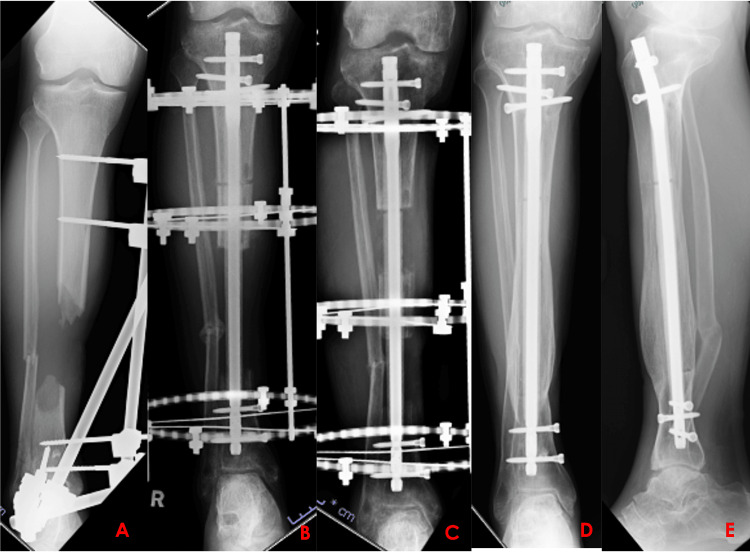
Serial radiographs of a case of bone transport over nail. (A) A trauma film shows significant bone loss of the tibia diaphysis. (B) Following an unhealthy bone resection, the defect is 10 cm. Locked intramedullary nail and ring fixator is performed with proximal corticotomy for transport over the nail. (C) Radiograph shows the bone transport segment being transported midway over the nail. (D and E) Radiographs show docking site union and transport segment consolidation at 10 months.
